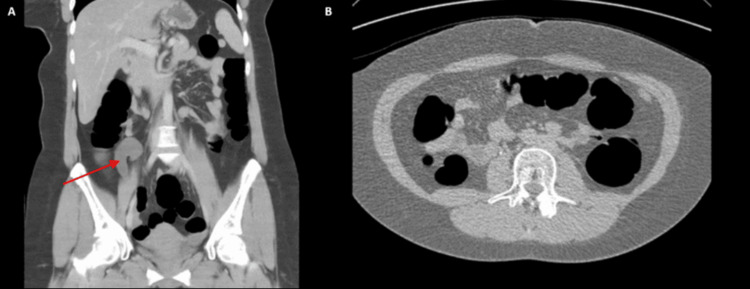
Computed tomography colonography (Case 3). (A) Coronal section with the red arrow indicating an apparent mucocele of the distal two-thirds of the appendix, measuring approximately 8 cm in length and 2.5 cm in diameter; (B) axial section.
